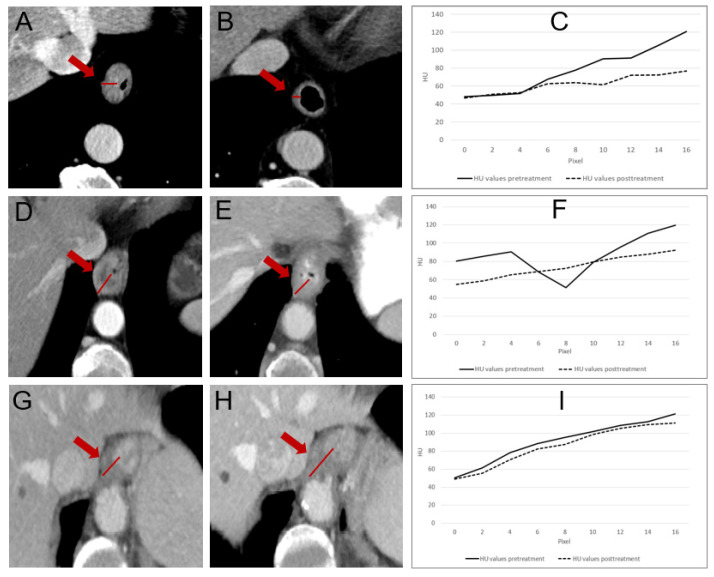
(A) Conventional iodine-enhanced CT in a patient diagnosed with type I adenocarcinoma of the GEJ. A line profile was set in the tumor region, with maximum HU value of 120.69 and density delta value of 69.05. (B) The same patient’s CE-CT after neoadjuvant treatment (grading of regression 1b). A line profile was again set in the prior tumor area, showing a maximum HU value of 76.65 and a density delta value of 24.19. (C) Plot profile showing a significant posttreatment HU value drop. (D,E) Iodine-enhanced CT scans in a patient with type I adenocarcinoma of the GEJ (grading of regression 2). Maximum pretreatment HU value is 119.87, maximum posttreatment HU value is 91.25. (F) Corresponding plot profile. (G,H) Line profiles in the tumor region of another non-responding type I adenocarcinoma of the GEJ patient (grading of regression 3). The maximum pretreatment HU value in G is 121.48 and posttreatment HU in (H) is 112.36. (I) Plot profile with no significant drop after neoadjuvant therapy.
